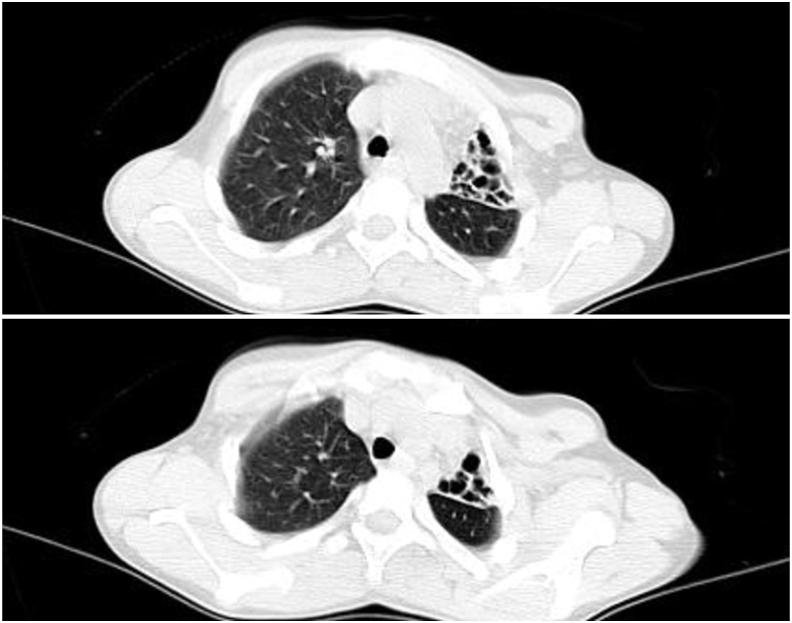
Patient's CT scan: multiple cystic-like lesions in the upper lobe of the left lung.
